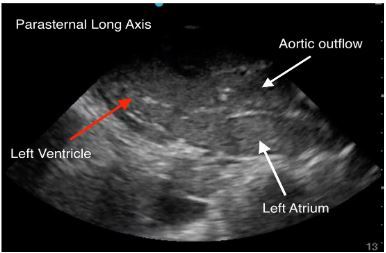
Transthoracic echocardiography parasternal long view of the heart demonstrating echogenic (thrombosed) blood within the left atria, left ventricle, and aortic outflow.
